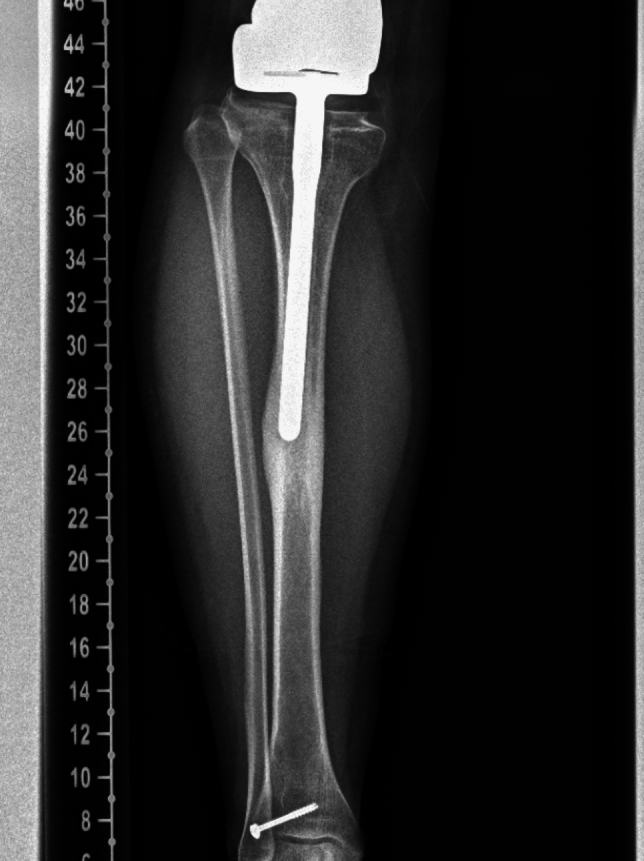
An 18 year-old female, eight years following expandable distal femoral endoprosthesis reconstruction, demonstrating marked cortical hypertrophy, progressive stem migration with varus deformity, and periosteal reaction on plain radiographs
